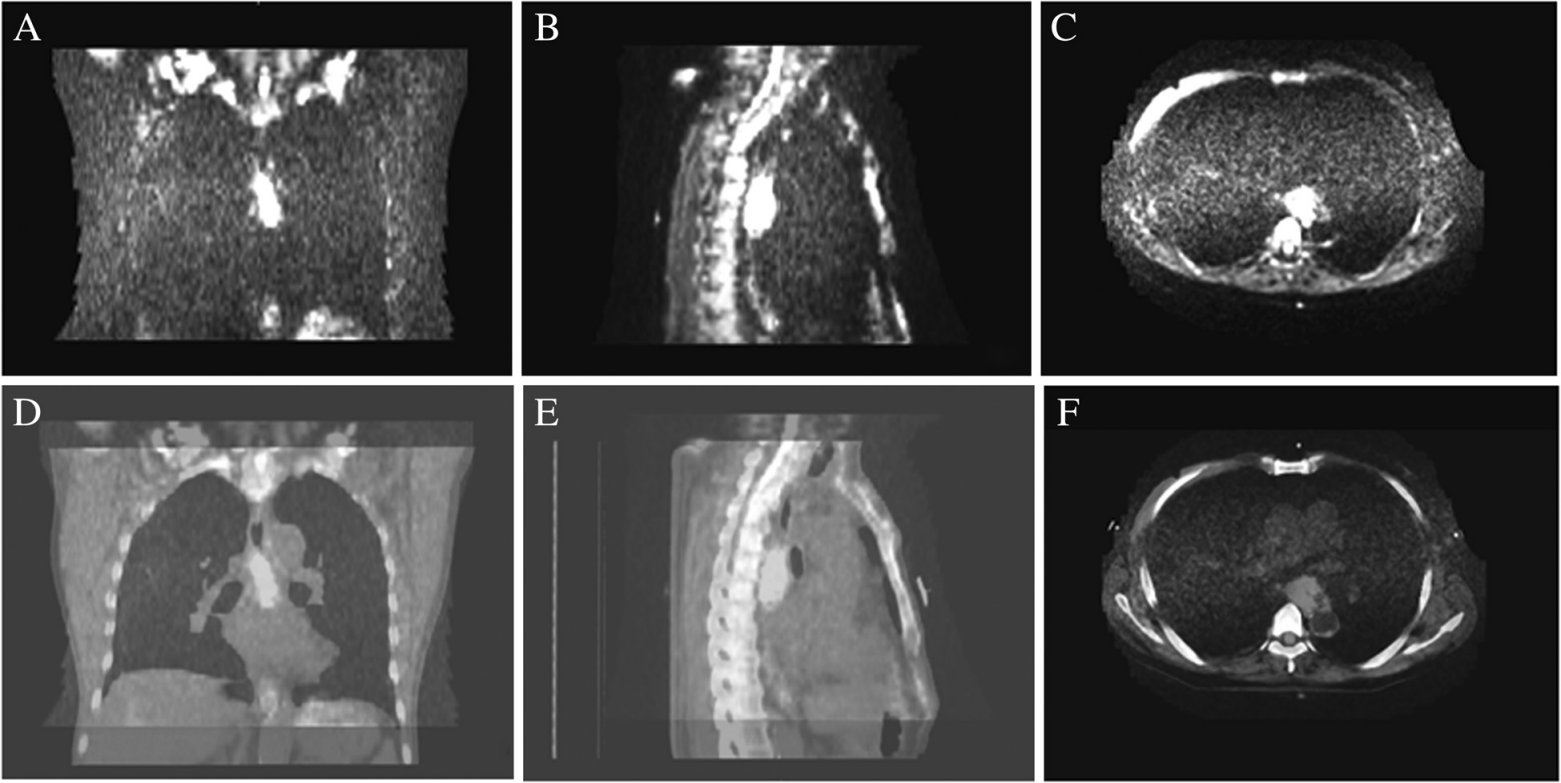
DWI scans and fused images. A, B and C show the coronal, sagittal and transverse images of DWI scans; D, E and F show the coronal, sagittal and transverse images of fused images.
